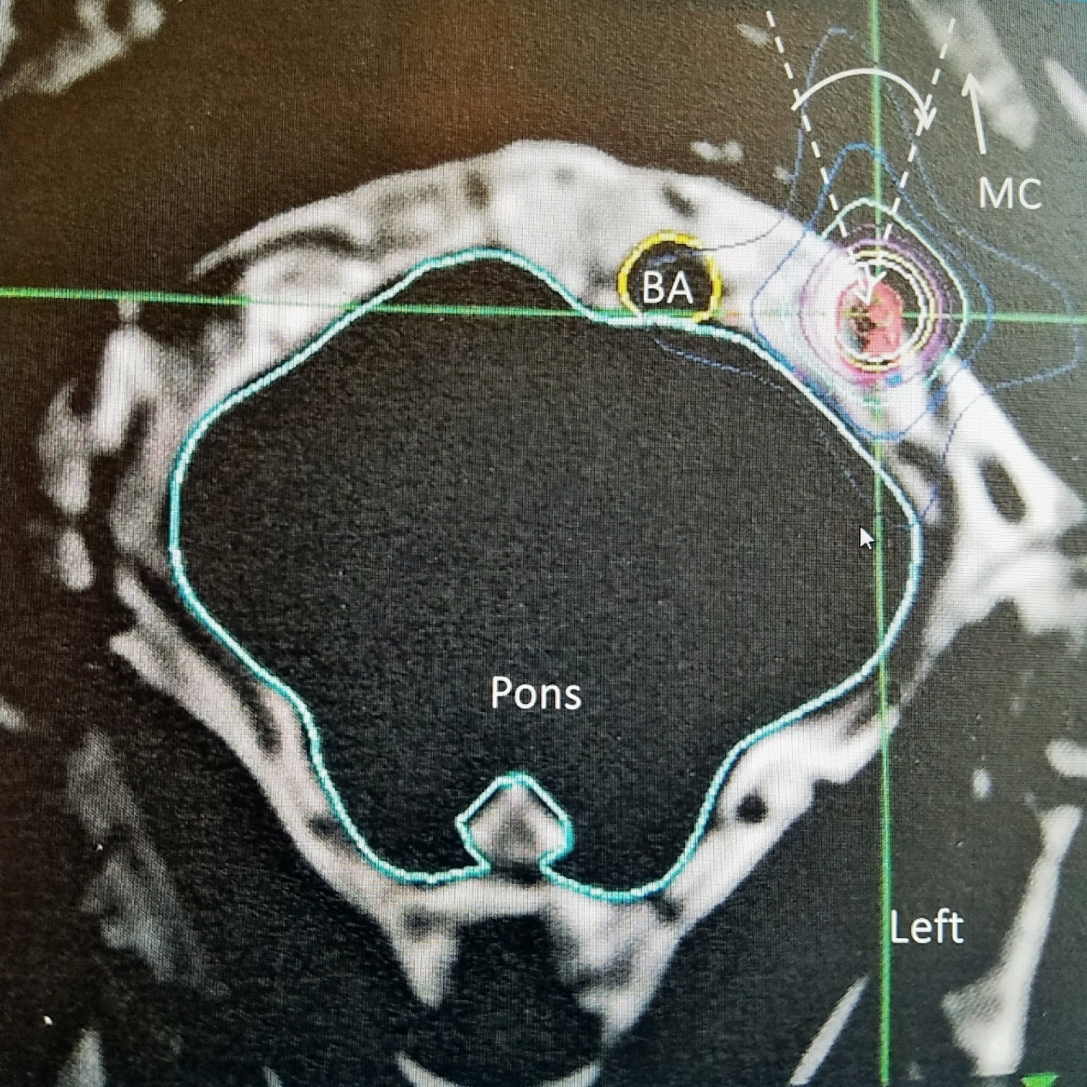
Axial T2 magnetic resonance image of stereotactic target to the left trigeminal nerve The 5 mm collimated target is aligned longitudinally along the axis of the trigeminal nerve. There is minimal edging along the brainstem and root entry zone. The basilar artery (BA) is identified as well as the mandibular condyle (MC). The dotted angle with the solid white arc is the spillover of beams toward the posterior left mandible where the oral carcinoma was found four years after the second stereotactic radiosurgery.
